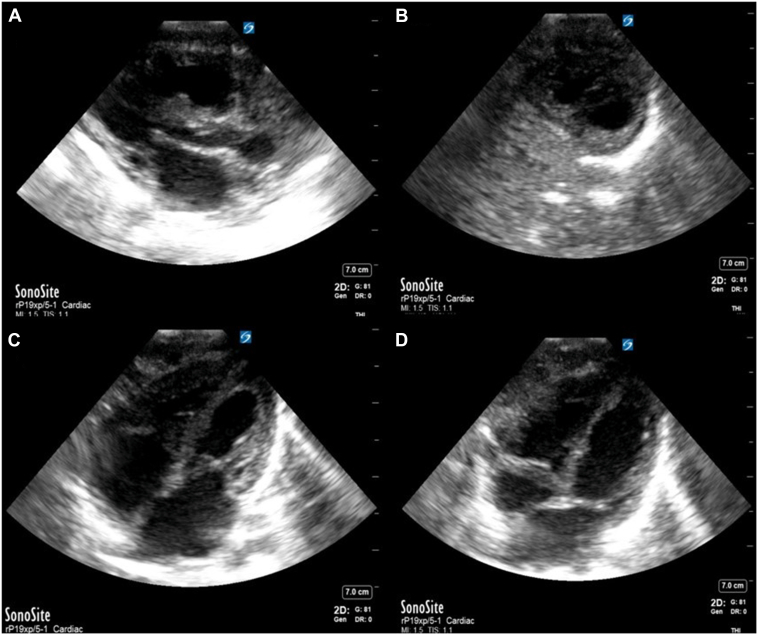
Standard (noncolor flow) images obtained during bedside point-of-care ultrasound echocardiogram performed in the emergency department. No significant abnormalities were noted in the parasternal long axis view (A), parasternal short axis view (B), apical 4-chamber cardiac view (C), or the subxiphoid cardiac view (D) using this imaging modality.
